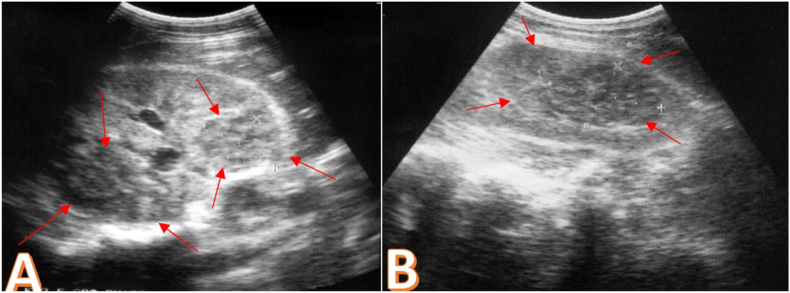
Renal ultrasound: multiple heterogeneous uncollected echogenic formations. A. Right kidney. B. Left kidney.
